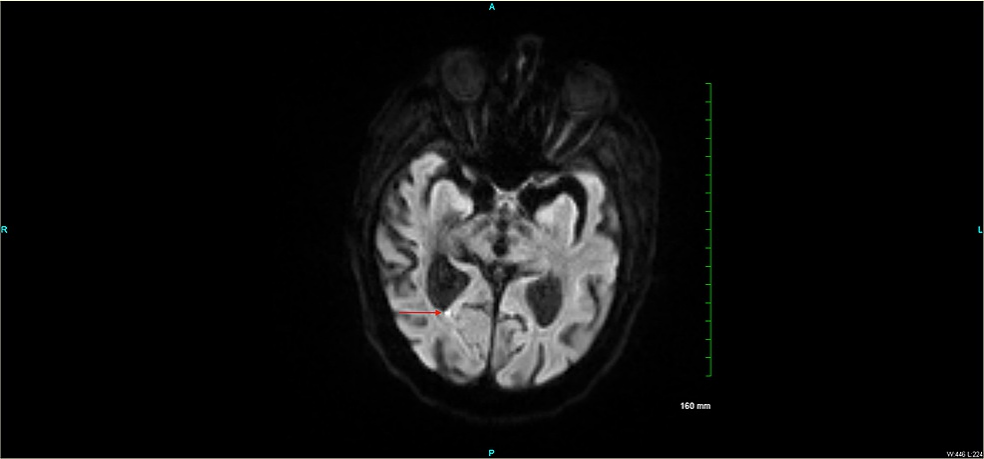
MR axial DWI sequence showing focus of restricted diffusion in the right splenial region of the corpus callosum. MR = magnetic resonance DWI = diffusion weight imaging
